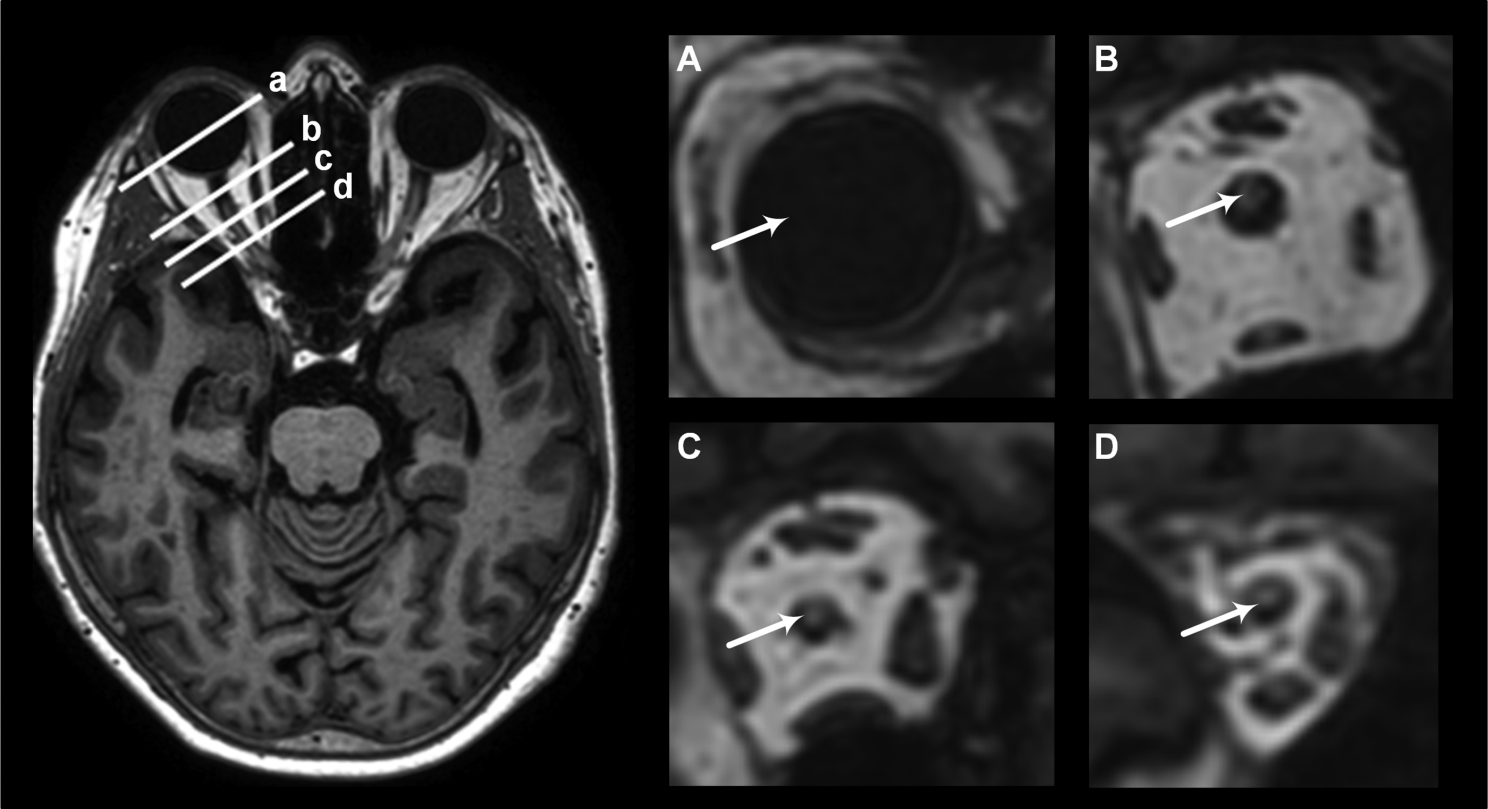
Anatomic location for placement of ROIs in the intraorbital segment of the visual pathway in iNPH using T1-MRI. The figure demonstrates the approximate intraorbital location along the visual pathway used for placement of the ROI. The left image provides an anatomical overview in the axial plane where the letters a–d correspond with the magnified coronal slices A–D, shown in the images to the right. The following locations are shown and indicated by *arrows*: Vitreous body (a/A), retrobulbar part of optic nerve (b/B), mid part of optic nerve (c/C), and posterior part of optic nerve (d/D).
